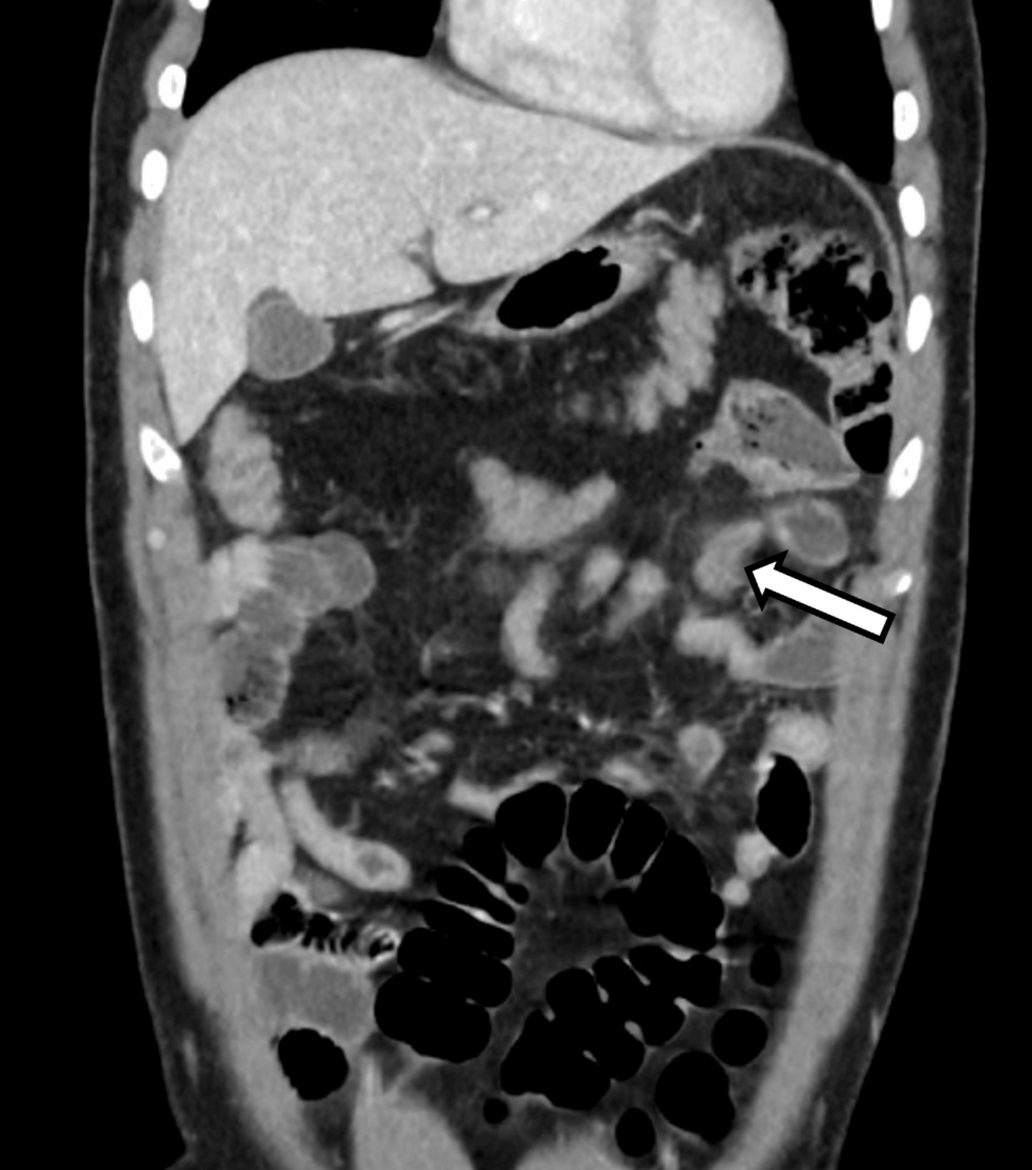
Inflamed appendix in the left upper quadrant Coronal CT image demonstrating the appendix abnormally located in the left upper quadrant, appearing distended with surrounding periappendiceal fat stranding, consistent with acute appendicitis. CT, computed tomography.
